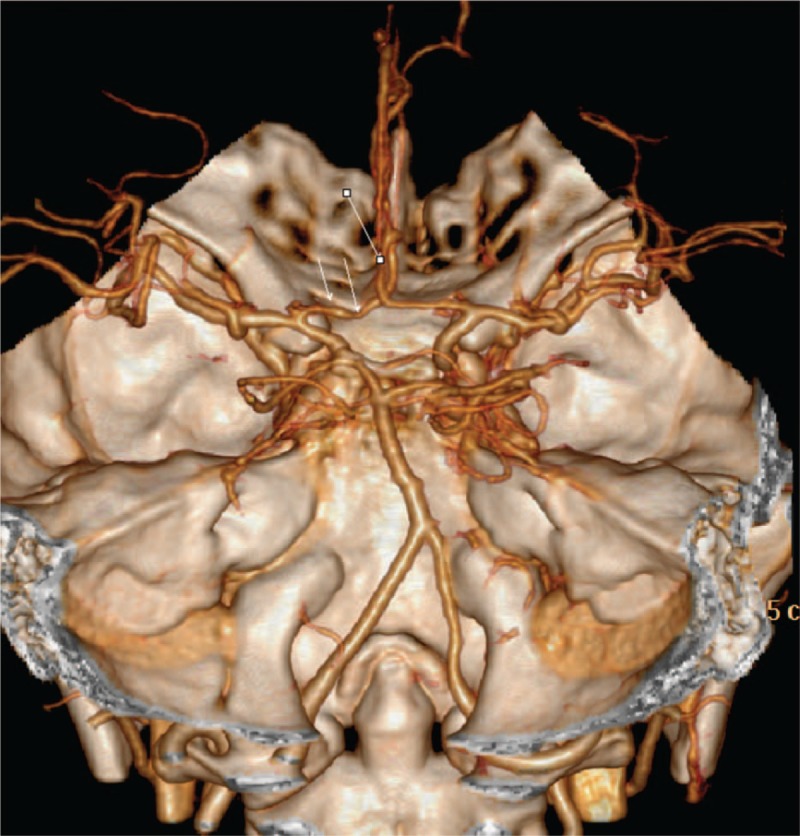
Head and Neck Computed Tomography Angiography. It showed bilateral, multifocal, reversible vasoconstriction of the anterior cerebral artery, leading to a diagnosis of RCVS.
